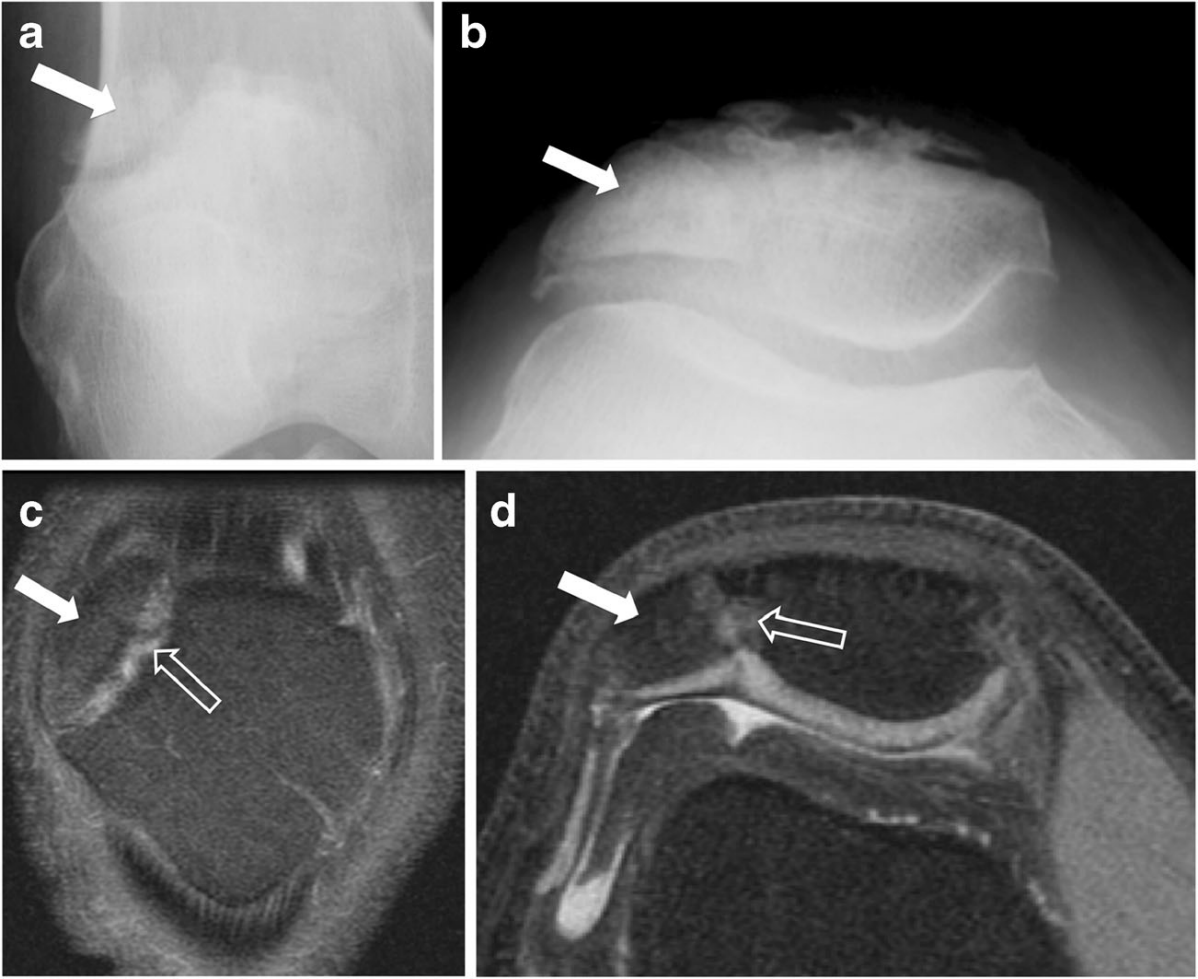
Two examples of incidental bipartite patella. (a) Anteroposterior and (b) tangential patellar radiographs of the right knee in the first example show a patellar fragment at the superolateral aspect of the right patella consistent with bipartite patella. In the second, (c) axial and (d) coronal fat-suppressed proton density MR images of the right knee demonstrate a well-corticated osseous fragment (*solid arrow*) at the superolateral aspect of the patella. Note the high signal of the synchondrosis (*open arrows*), the well-preserved underlying cartilage of the patella, and the lack of bone marrow oedema at the adjacent bone that differentiate this variant from a patellar fracture
